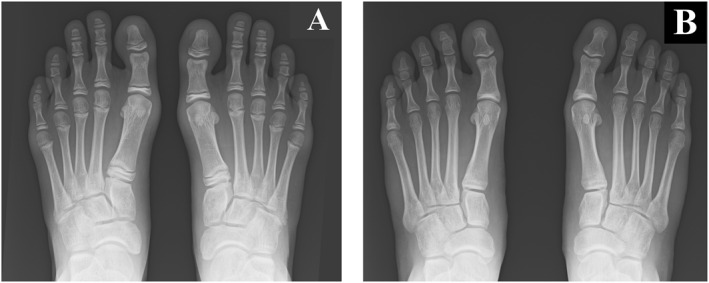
Representative weight‐bearing dorsoplantar radiographs demonstrating skeletal maturity stages. (A) A 13‐year‐old male with open physes at the base of the first metatarsal and proximal phalanx. (B) A 14‐year‐old female with closed physes at the corresponding sites.
